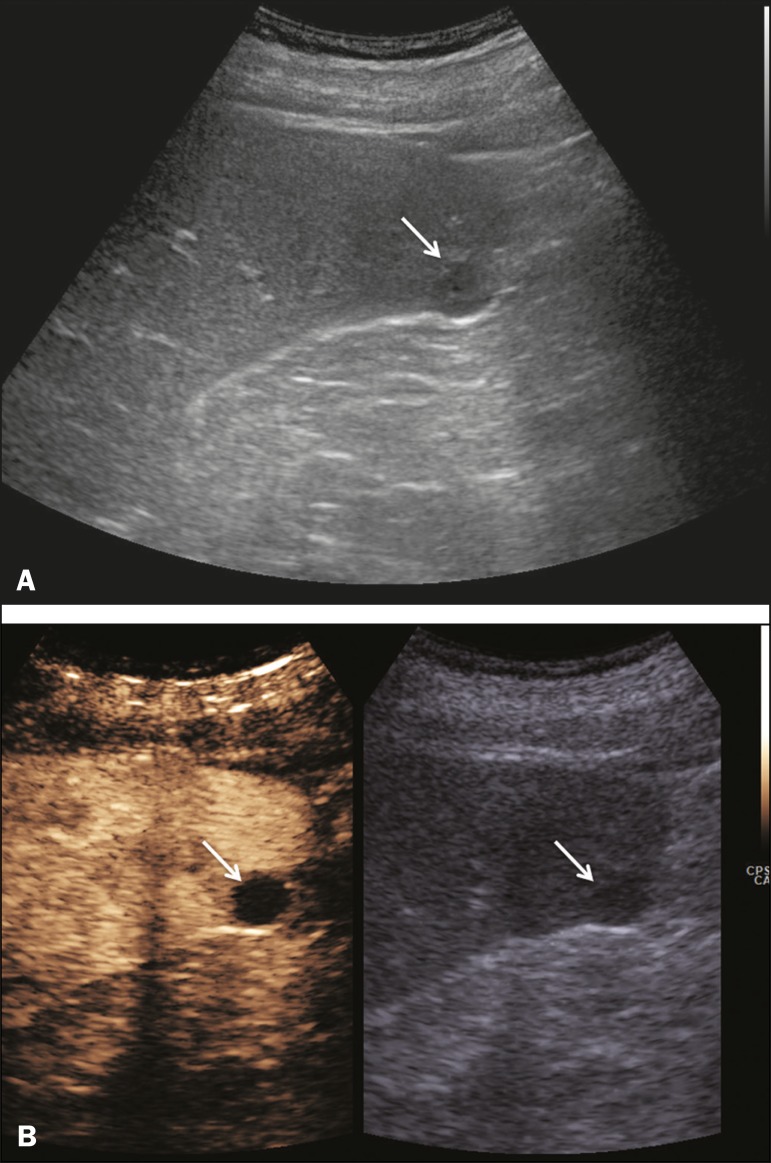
Simple cyst. A: B-mode ultrasound showing a
